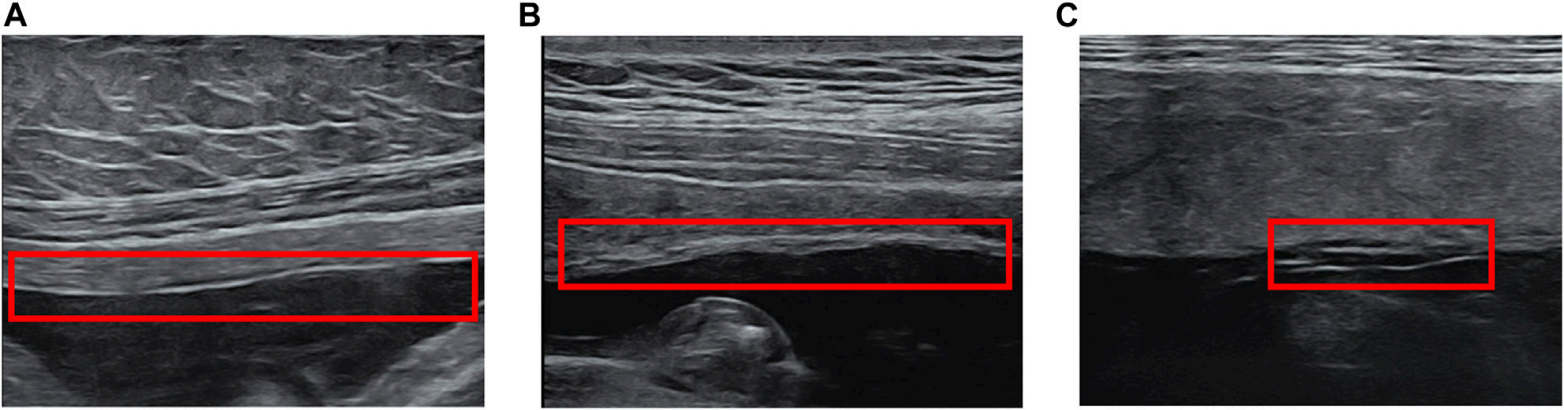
Fetal membrane abnormalities in ultrasound. (A) Normal FM (B) FM Thickening (C) Chorioamniotic Separation.
